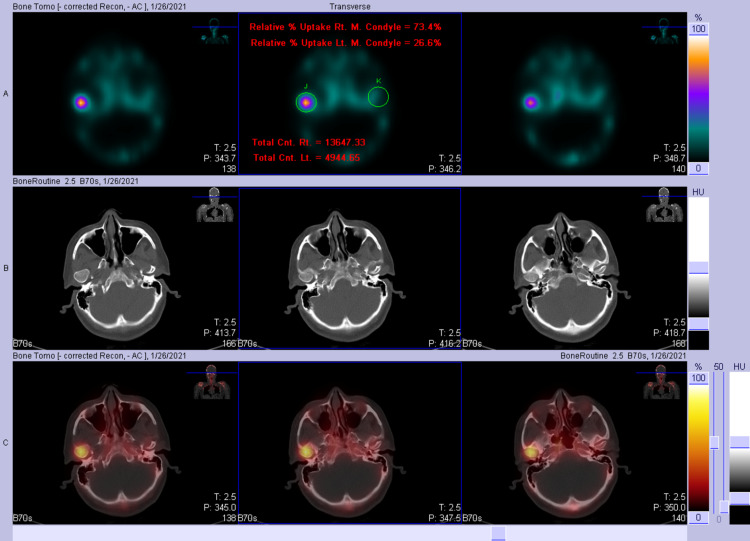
SPECT image demonstrating intense focal activity at the right mandibular condyle and normal physiological tracer uptake at the left mandibular condyle. SPECT: single-photon emission computed tomography
